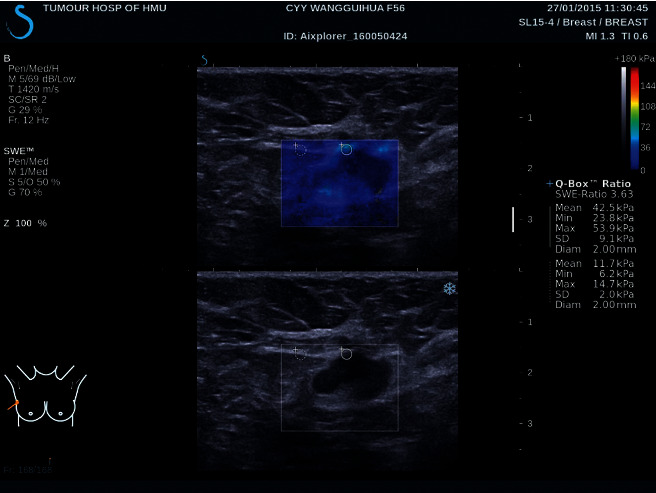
Shear wave elastography (SWE) and B-mode ultrasonography (BUS) images of a metastatic axillary lymph node from an invasive breast cancer in a 56-year-old women. Findings consistent with malignancy on BUS included loss of hilar fat and cortical heterogeneous echogenicity (lower image). SWE was performed for the lymph node and a Q-box is placed over the stiffest region, as assessed by visual inspection. A 2-mm-size circular region of interest was selected at the stiffest portion and elasticity scores of 42.5 kPa (*E*_max_), 53.9 kPa (*E*_mean_), 23.8 kPa (*E*_min_), and 3.63 (*E*_mean-m_)were obtained. *E*_max,_ maximal elasticity score of the lesion; *E*_mean,_ mean elasticity score of the lesion; *E*_min,_ minimal elasticity score of the lesion; *E*_mean-m,_ ratio of *E*_mean_ mean of the lesions divided by *E*_mean_ of surrounding muscles.
